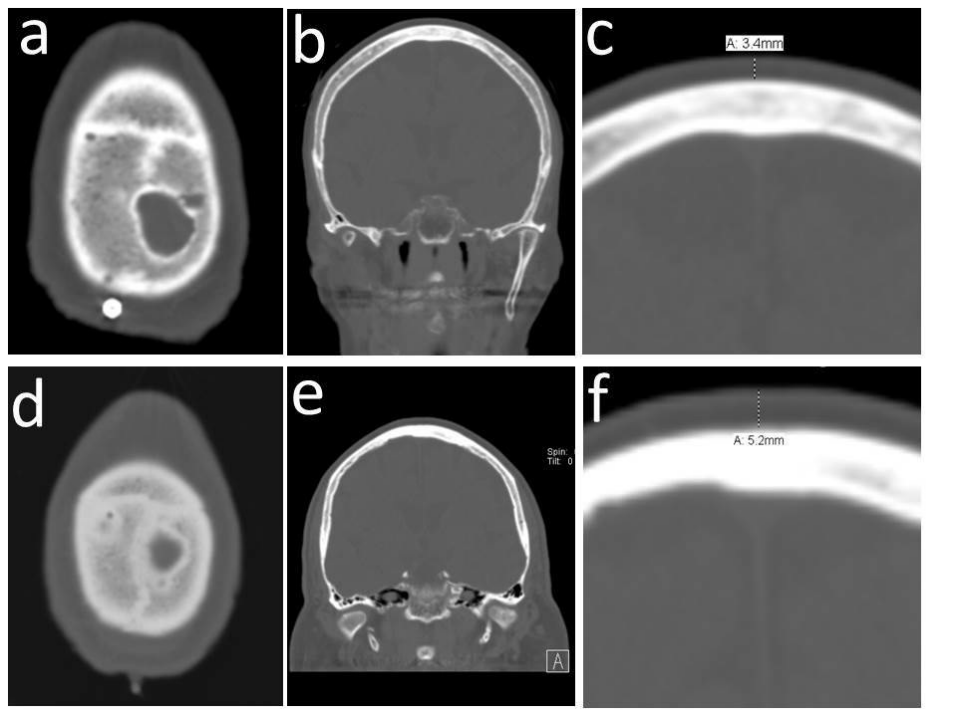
Measurement of Scalp Thickness in Two Patients (a-c and d-f) a,d: Axial CT scans showing typical coronal suture imaging. b, e: Coronal CT reconstructions at the level of the coronal suture for both patients. c, f: Examples of the measurement of scalp thickness at the midline over the coronal suture.
